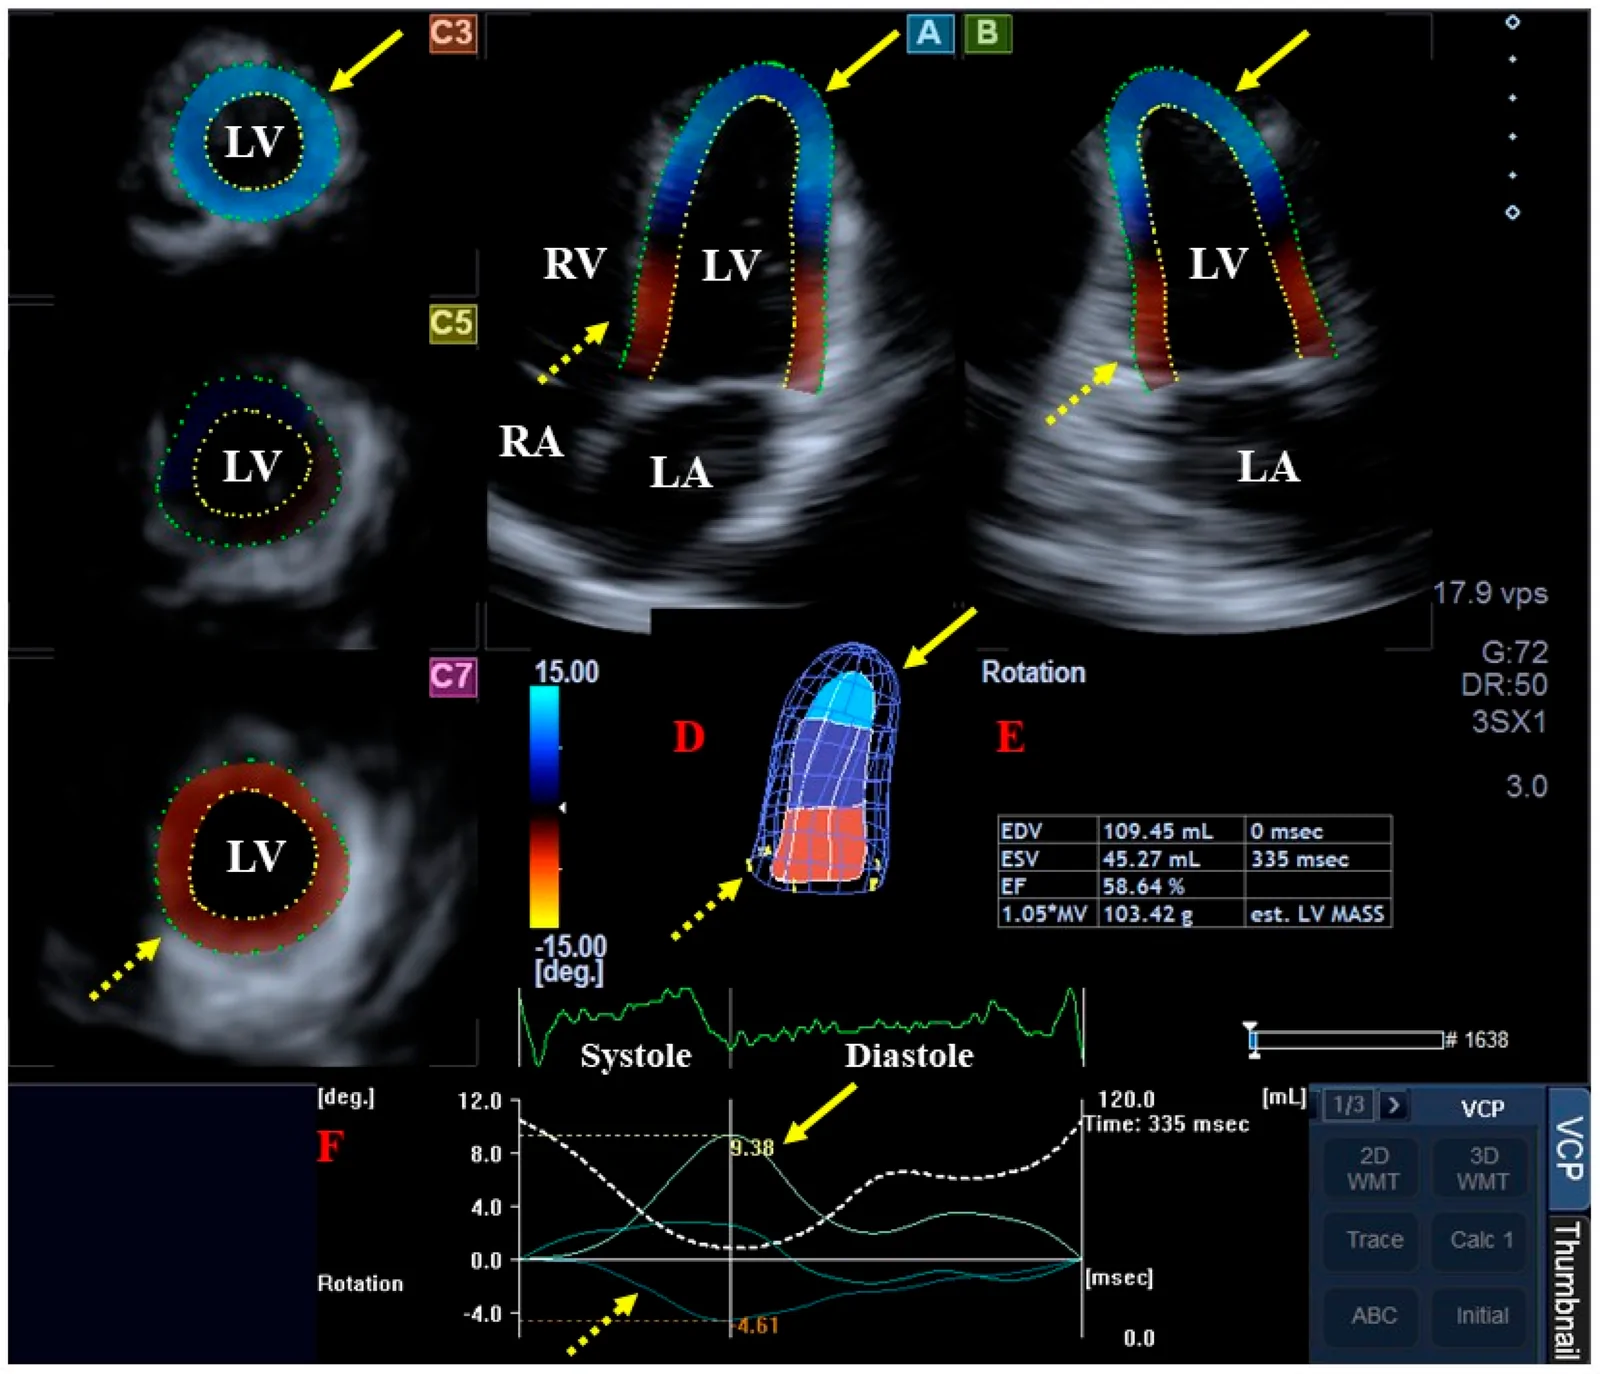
Assessment of left ventricular (LV) rotational parameters using three-dimensional (3D) speckle-tracking echocardiography. Representative apical four-chamber (A) and two-chamber (B) long-axis views are shown, along with short-axis views at the apical (C3), midventricular (C5), and basal (C7) levels. A virtual 3D reconstructed model of the LV (D) and calculated LV volumetric data (E) are also displayed. Apical (solid yellow arrow) and basal (dashed yellow arrow) LV regions and their time-LV rotational curves are presented, together with a curve representing temporal LV volume changes (dashed white line) throughout the cardiac cycle (F). Abbreviations. LV = left ventricle, LA = left atrium, RV = right ventricle, RA = right atrium.
